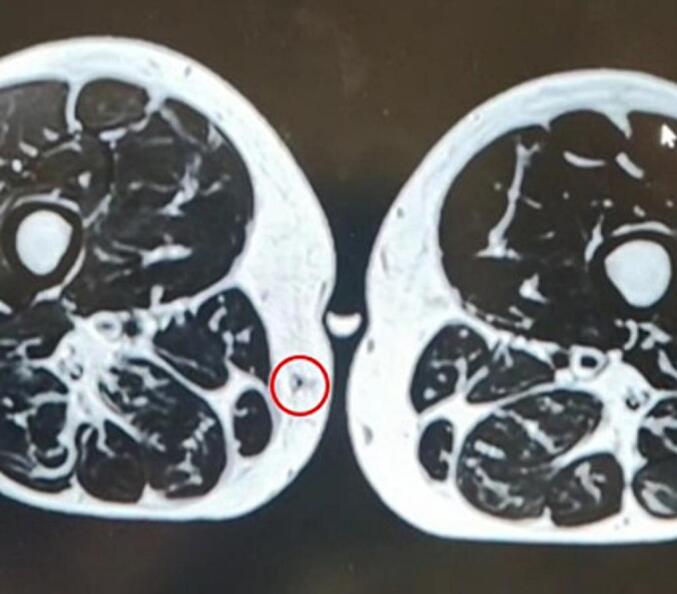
Thigh MRI with gadolinium, T1 sequence, showing a 4 × 2 mm hypointense, oval-shaped nodule (Red circle) located in the subcutaneous area far from the dermis on the median right thigh. (For interpretation of the references to colour in this figure legend, the reader is referred to the web version of this article.)
